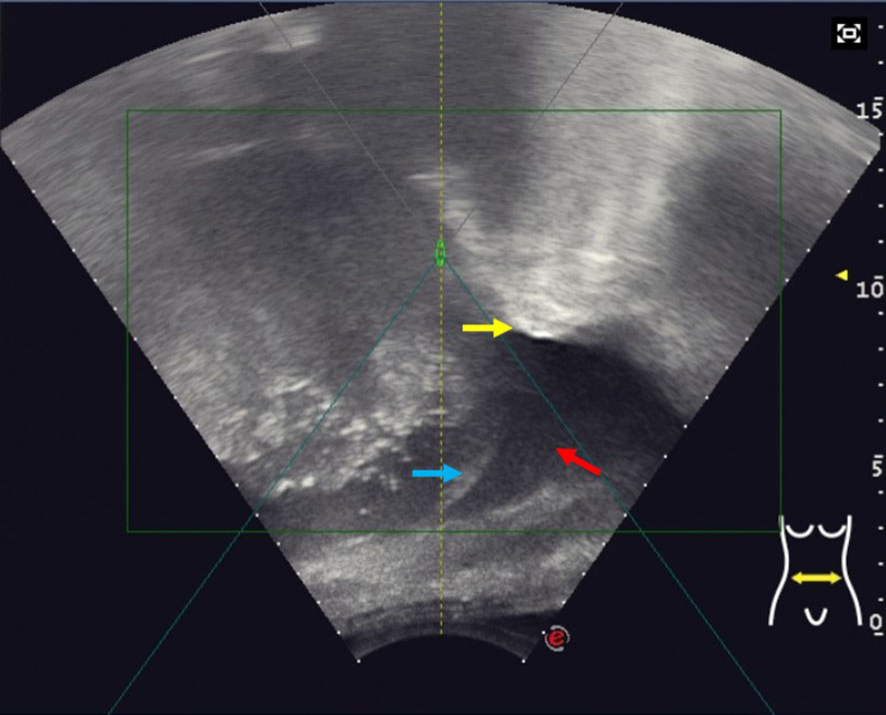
Representative ultrasound image monitoring during HIFU showing a good ultrasound pathway to remove lung tissue using the artificial pleural effusion method. The yellow arrow indicates the lung pushed by artificial pleural fluid; The blue arrow shows the diaphragma; The red arrow shows the artificial pleural fluid (injected normal saline).
